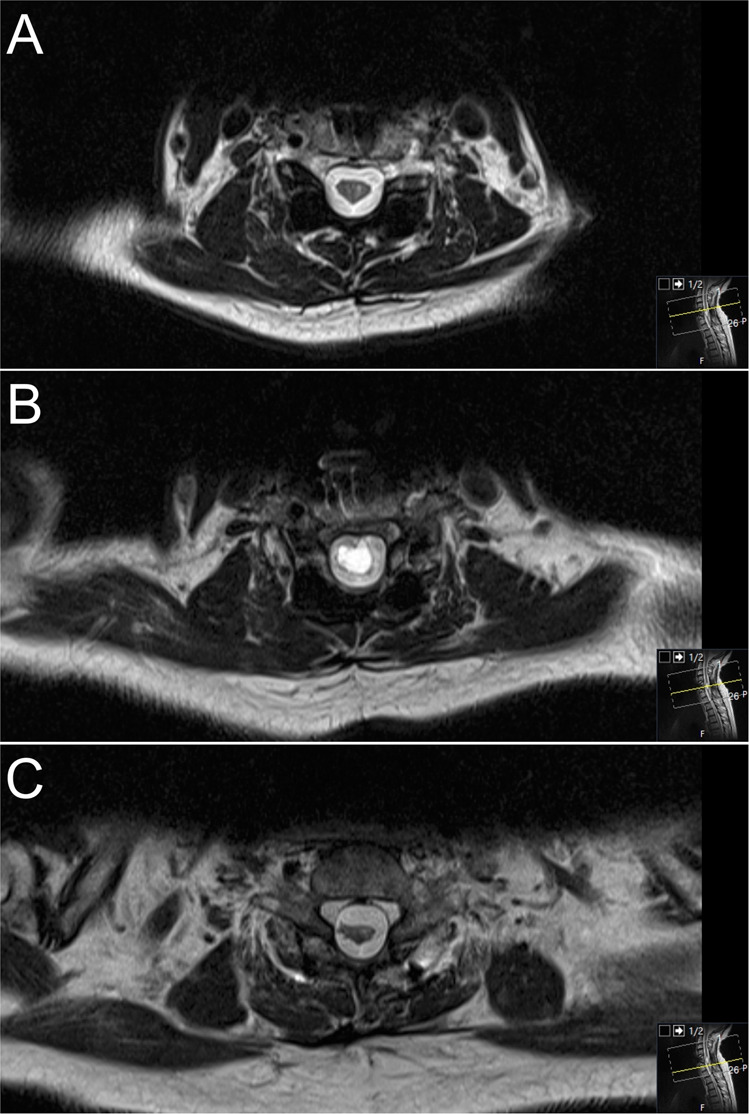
MRI of posttraumatic tethered cord. Axial T2 magnetic resonance images showing a posttraumatic tethered cord with syrinx formation above the cyst (A), in level with the cyst (B) and below the cyst (C).
